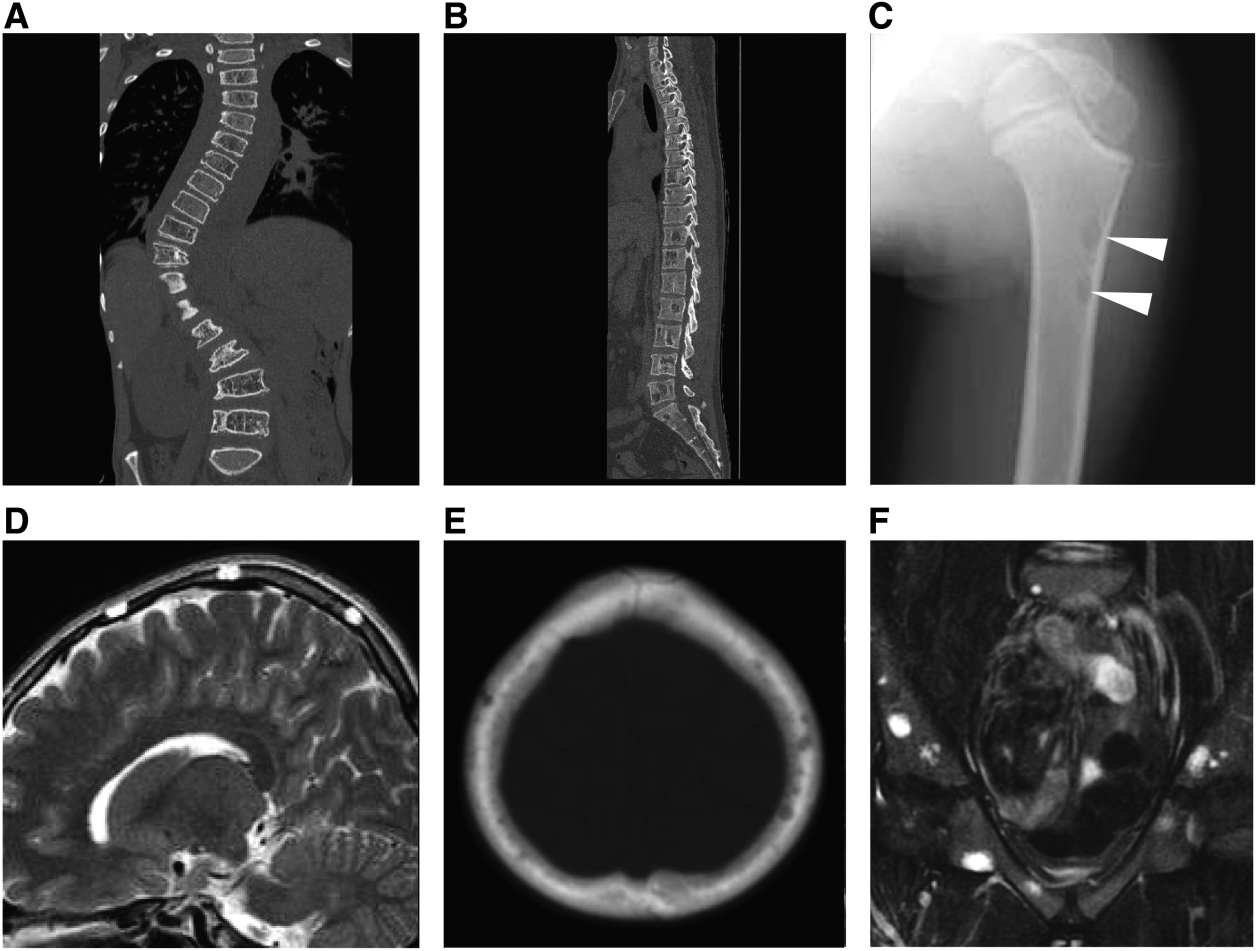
Radiological findings of patients with GLA and KLA. The multiple lytic areas in the medullary cavity are shown in the osteolytic images of GLA and KLA. (A) A 12-year-old male with KLA involving the region from the thoracic to lumbar spine. Disseminated osteolysis is shown in CT with coronal reconstruction of in the vertebrae. The patient complained of severe pain, which was treated with opioid. It was difficult to operate to treat his scoliosis because of severe thrombocytopenia and bone fragility. (B) A 31-year-old male with GLA involving the region from the thoracic to lumbar spine. He did not complain of any symptoms. (C–F) An 8-year-old male with KLA involving multiple bone lesions. He did not also complain of any symptoms. (C) A small lytic lesion is shown in plain radiography of femoral head (*white arrowheads*). (D, E) Sagittal and axial T2-weighted MRI and CT scans of the head show a small lytic lesion. (F) Multiple cystic lesions are shown in a coronal fat-saturated T2-weighted MRI of the pelvis. KLA, kaposiform lymphangiomatosis.
